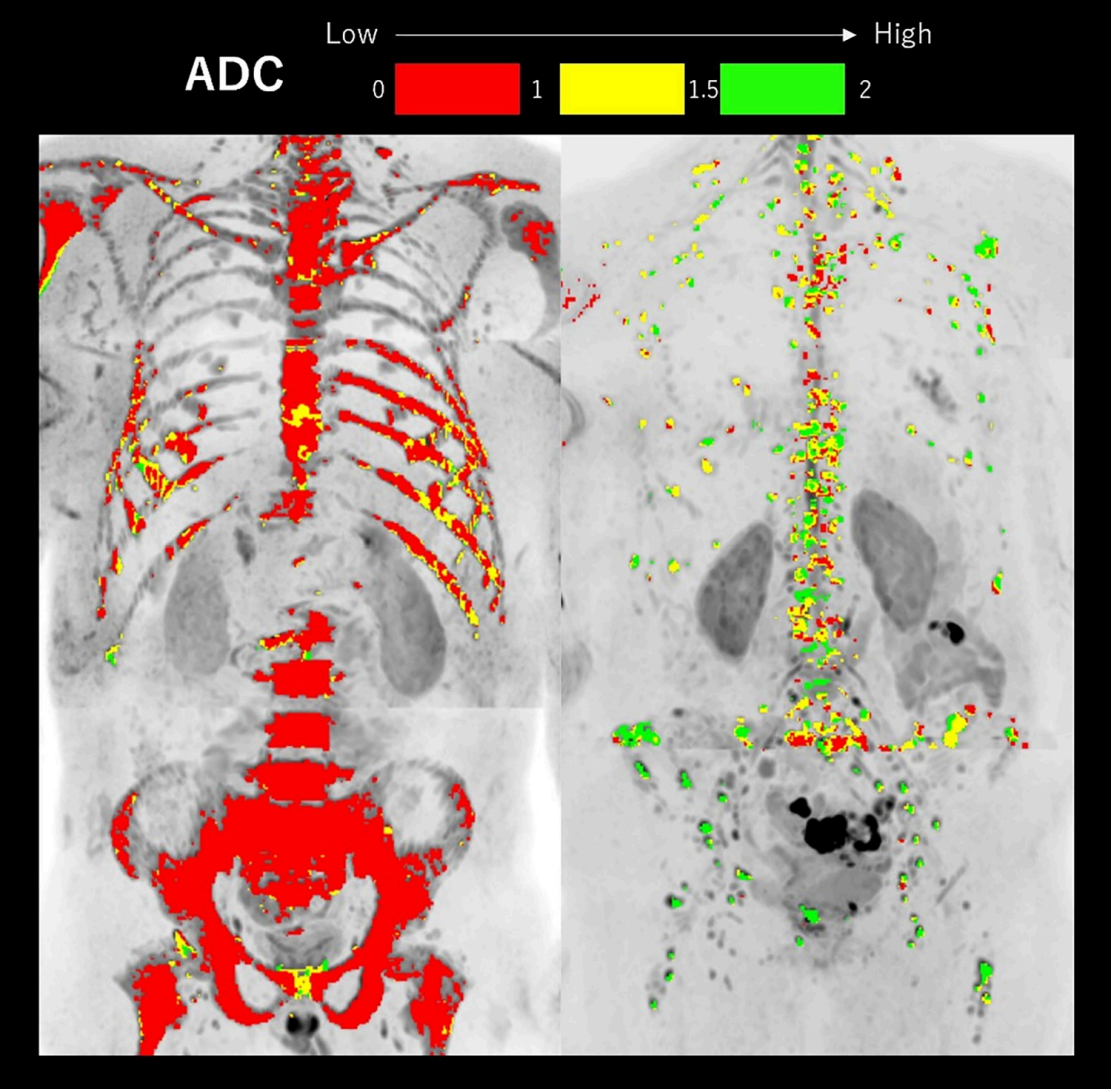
Representative whole-body diffusion-weighted imaging (DWI) for the patient classifications of mobilizer and relatively poor mobilizer. Left image, mobilizer: Whole-body DWI of a 47-year-old man with symptomatic myeloma who had an adequate number of collected CD34^+^ cells (4.4×10^6^ CD34^+^ cells/kg). Median ADC of the total diffusion volume was 0.78×10^−3^ mm^2^/s. Right image, relatively poor mobilizer: Whole-body DWI of a 59-year-old woman with symptomatic myeloma who had an inadequate number of collected CD34^+^ cells (3.8×10^6^ CD34^+^ cells/kg). Median ADC of the total diffusion volume was 1.53×10^−3^ mm^2^/s.
